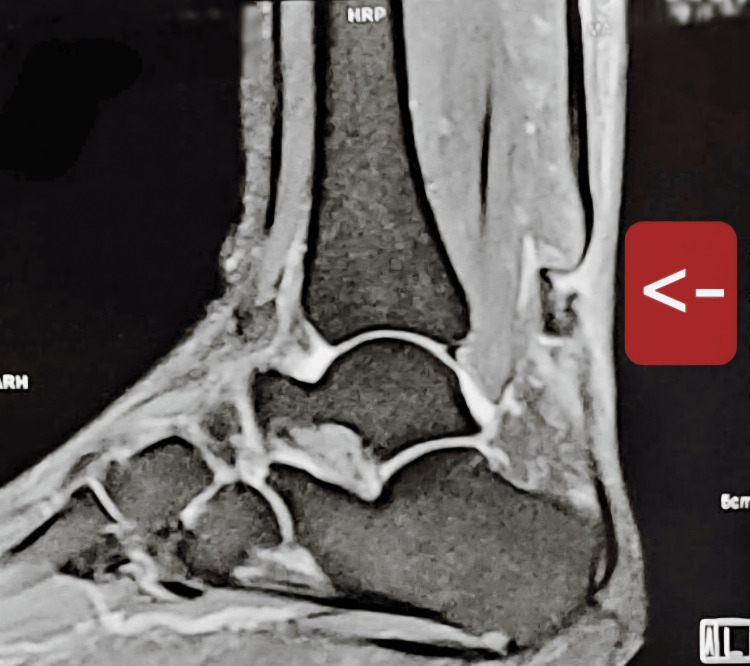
Title: Right Ankle MRI (Sagittal View) Demonstrating Chronic Achilles Tendon Rupture Sagittal MRI of the right ankle demonstrating complete discontinuity of the Achilles tendon (arrow) with proximal tendon retraction and interposed degenerative tissue, consistent with a chronic rupture. The tendon defect (gap size) is estimated to exceed 5 cm, supporting the need for reconstructive management rather than primary repair.
